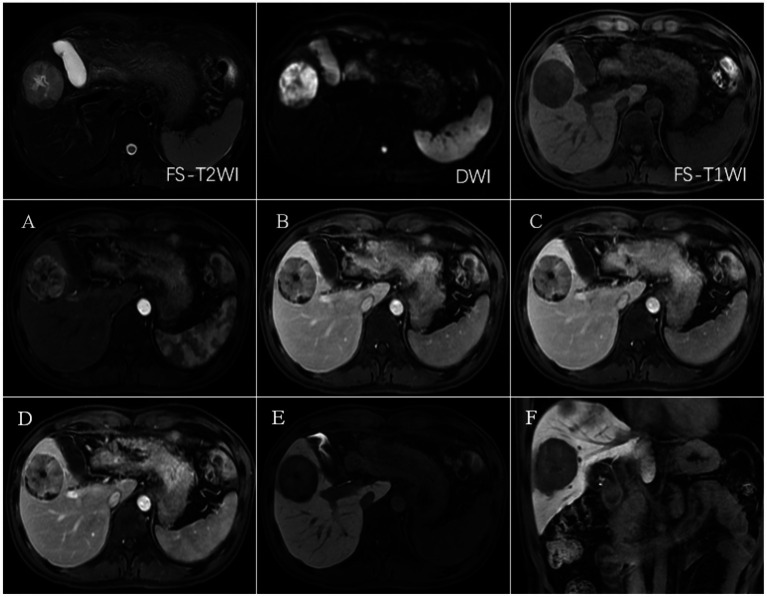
FS-T2WI and DWI: rounded high signal in the right lobe of the liver, with a diameter of about 48.9 mm; FS-T1WI: rounded low signal in the right lobe of the liver. (A–F) show the early arterial, late arterial, portal venous, delayed, hepatobiliary axial and coronal positions. EOB-MRI enhancement scan can accurately show the enhancement of the lesion after TACE: the surviving area shows increased signals in T2WI and DWI, and the enhancement scan shows the characteristics of “fast-in-fast-out” enhancement, with low signals in the hepatobiliary phase. The surviving areas showed increased T2WI and DWI signals, with “fast-in-fast-out” enhancement characteristics and low signals in the hepatobiliary phase. The necrotic area showed high signal in T1WI, low signal in T2WI and DWI, no enhancement in the enhanced scan, low signal in the hepatobiliary phase, and coagulative necrosis.
